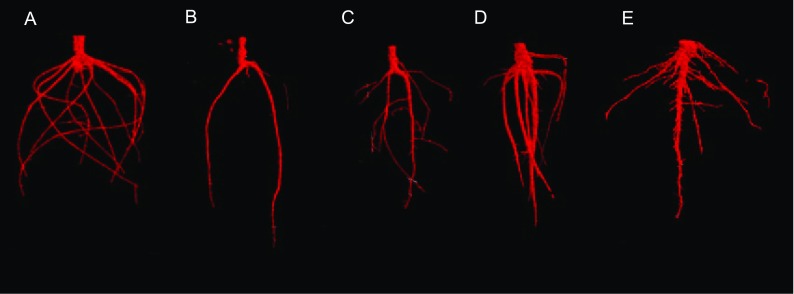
Selected images generated from 3D X-ray Computed Tomography. a CONT ‘tap and fibrous’ *Senecio jacobaea,*
